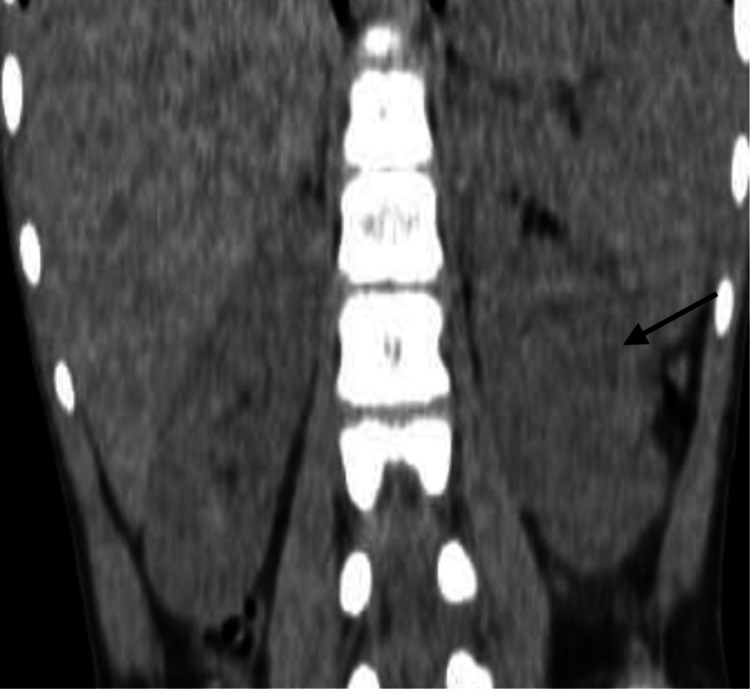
Coronal non-contrast CT of the left kidney showing a mid-polar lesion isodense to adjacent cortex (arrow)
